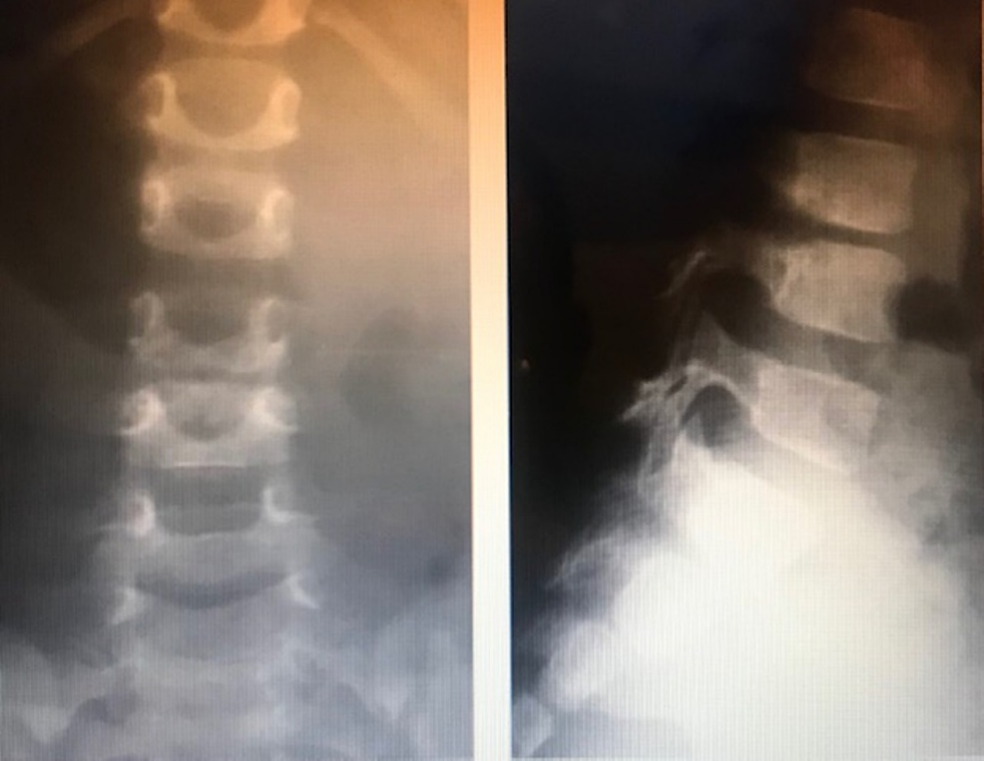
Lumbar spine x-ray of a three-year-old child with a two-week history of irritability, showing disk space narrowing of L3-L4 consistent with spondylodiscitis
